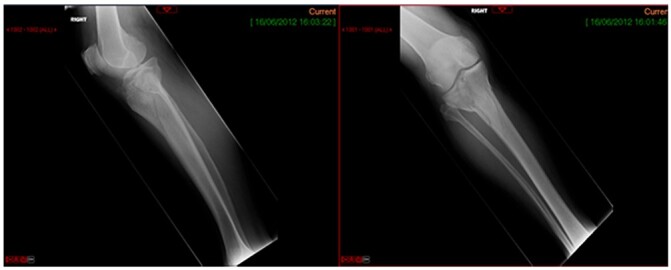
Plain radiograph AP and lateral.
